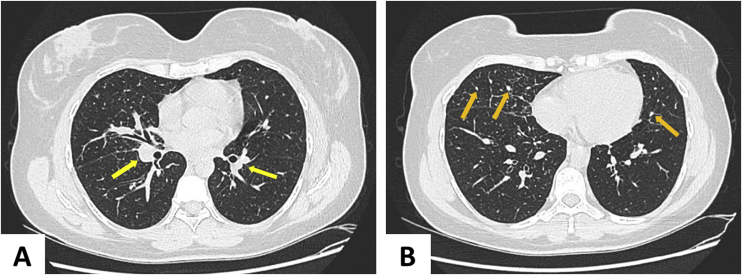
Computed tomography of the chest showing parabronchial nodules – yellow arrows (A) and multiple peripheral centrilobular micronodules – orange arrows (B).
